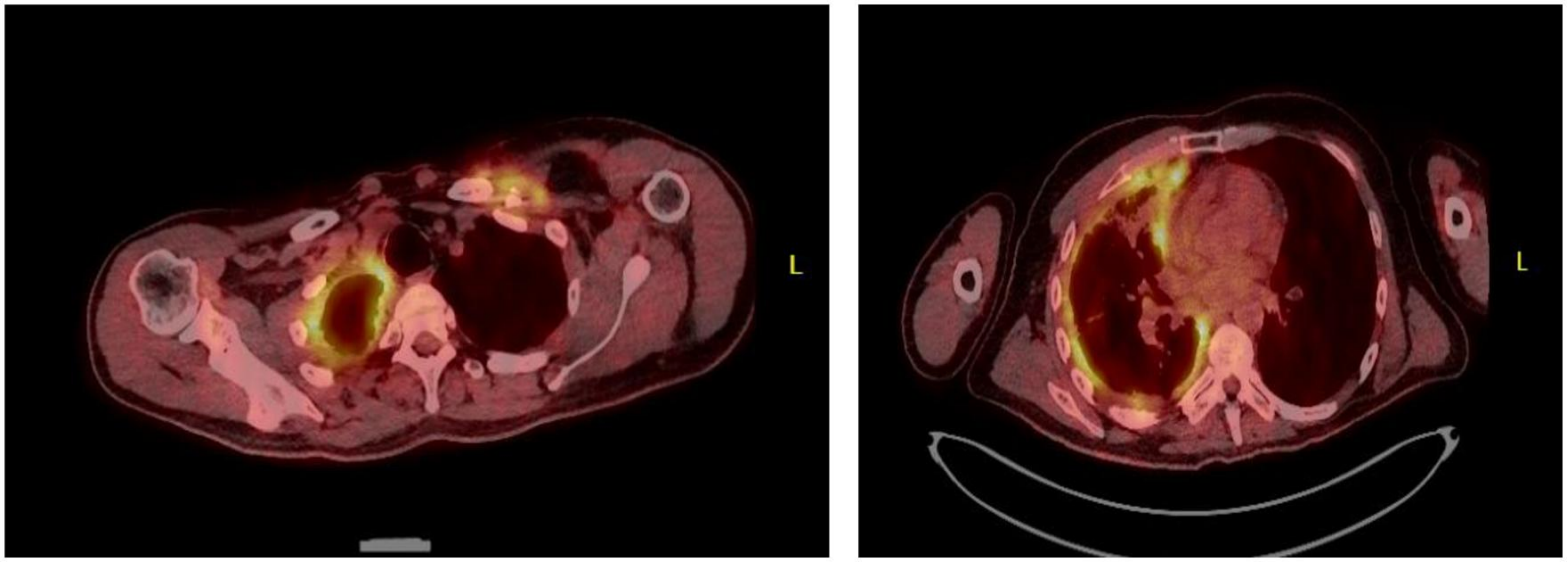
PET-CT Images Showing (A) an FDG-Avid Left Supraclavicular Mass (Arrow) and (B) Ring-Like FDG-Avid Right Pleural Thickening (Arrow) with Associated Atelectasis, Consistent with Metastatic Epithelioid Hemangioendothelioma
